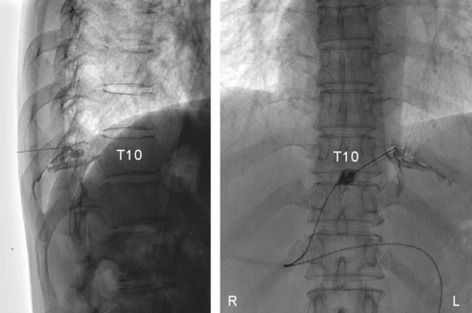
Thoracic X-ray images (*left*, lateral view; *right*, anterior-posterior view) during the left T10 intercostal nerve block. Contrast medium was injected to indicate the site of the nerve block
